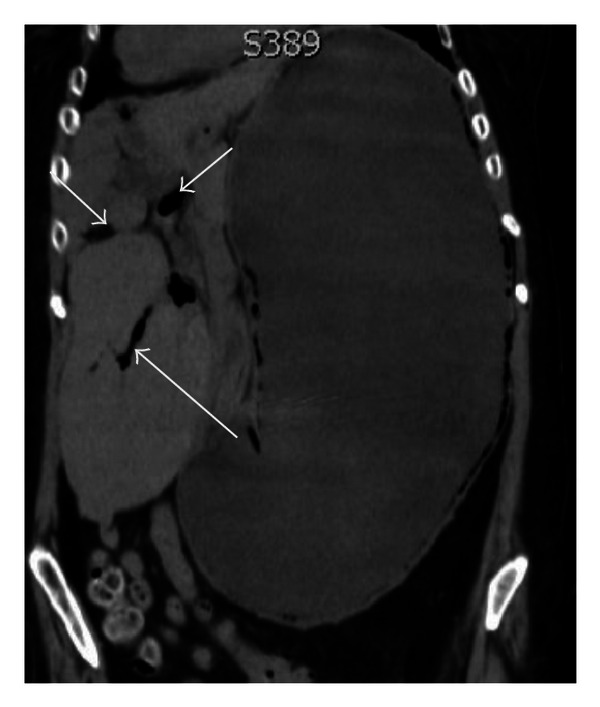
Portal venous gas on CT scan, coronal reformat (arrows).
